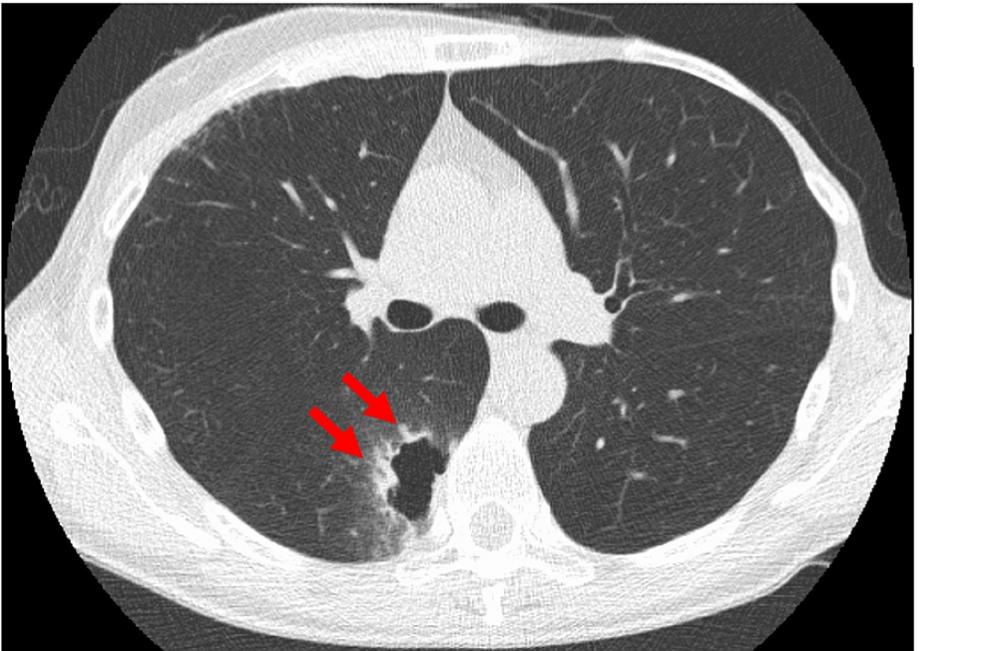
CT image at the time of diagnosis of right lung cancer This is the CT image of right lung cancer with a cavitation which is pointed by the arrows.
